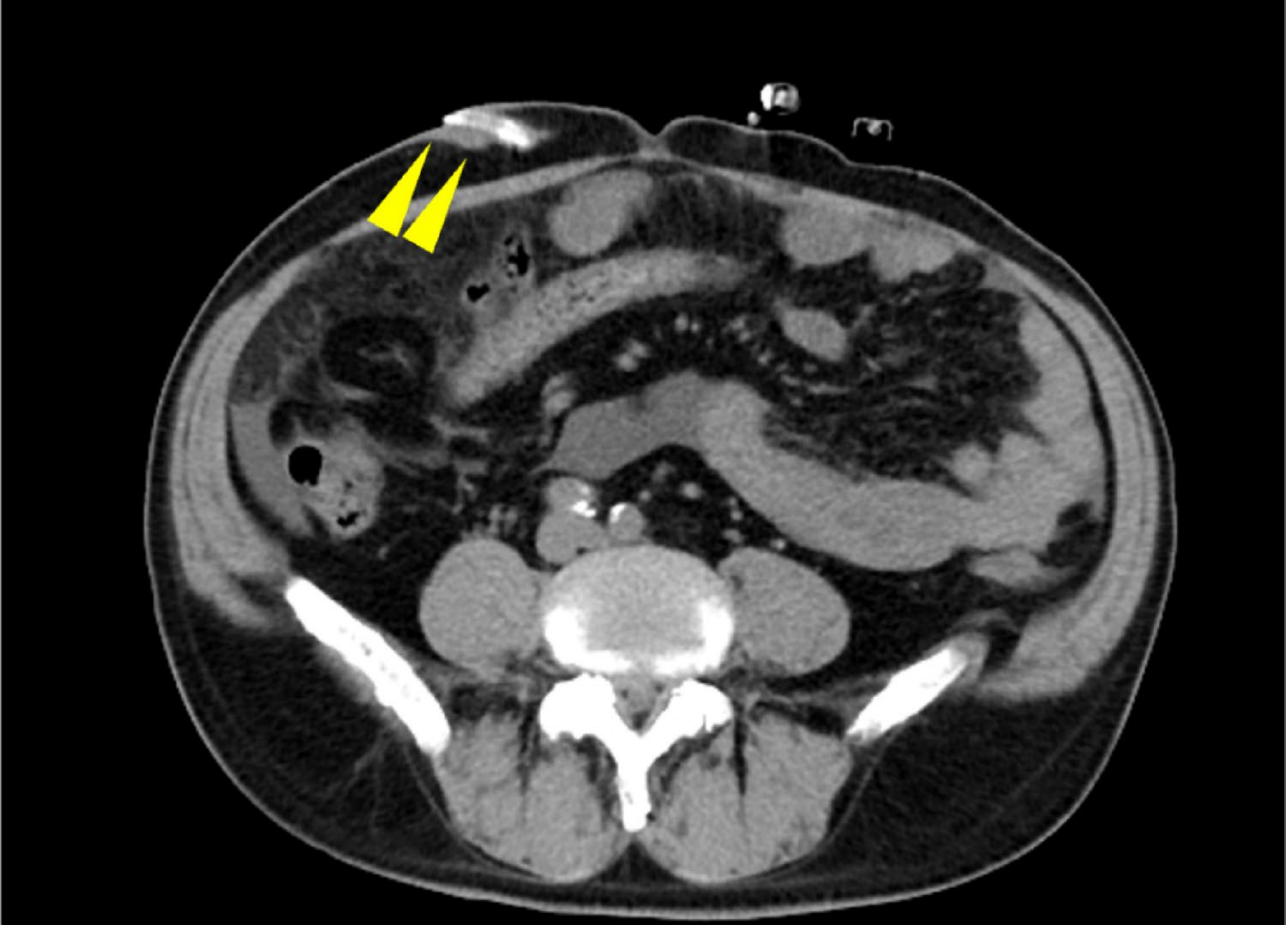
Plain computed tomography (CT) imaging of the second episode of peritonitis. The yellow arrows show chronic inflammation around the catheter near the external cuff
